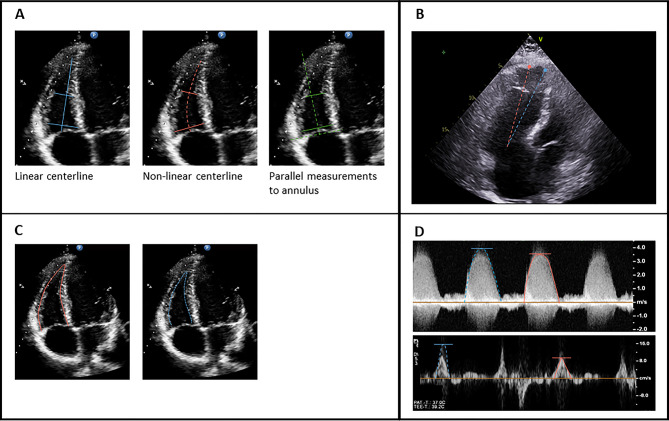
Echocardiographic images related to standardization of measurements - all of them were used in the survey. (A) Definition of centerline for transverse measurements. Left: Linear centerline (from mid annulus to RV apex; blue); Middle: A non-linear centerline that follows the curvature of the right ventricle (similar to what is done for the aorta or coronary arteries (red): Right: Parallel measures to the tricuspid annulus independent of the centerline (green). (B) Definition of RV apex: Blue dotted line: apex positioned at the septal junction; red dotted line: apex defined as the maximal distance from the right ventricular centroid to the RV contour. (C) Definition of endocardial/blood-myocardium border: left - at the compacted region (red); right – at the non-compacted region. (D) Use of modal frequency for Doppler measurements. Upper image: continuous-wave blood Doppler (red line: modal frequency; blue dotted line: maximal velocity). Lower image: tissue spectral Doppler (red line: modal frequency; blue dotted line: maximal velocity)
